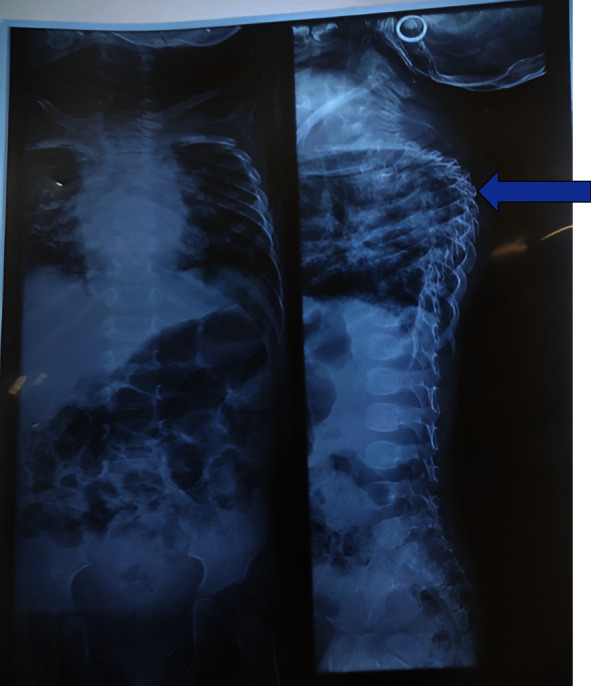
Chest X-ray showing diffuse infiltrates over the bilateral lung field and an X-ray of the spine showing kyphotic deformity of the thoracic vertebra (arrow).
